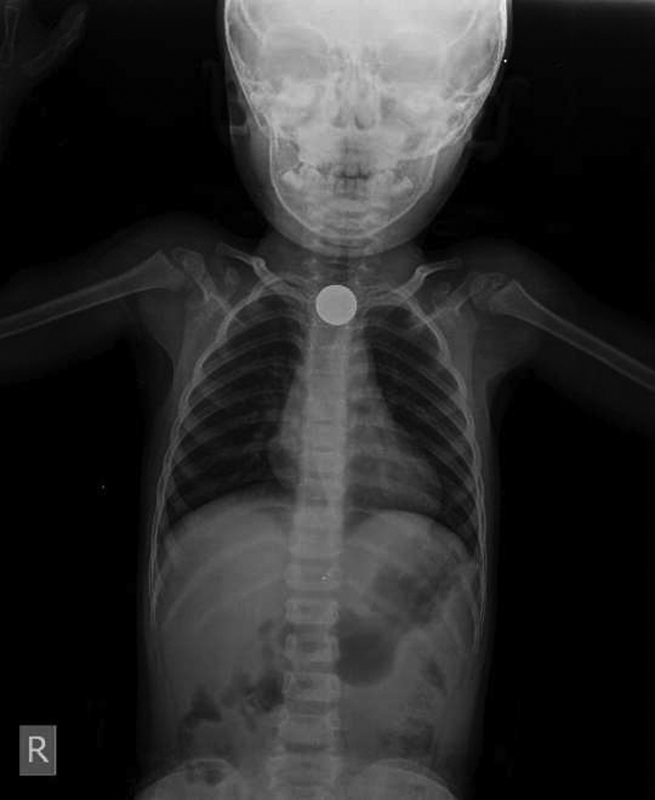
X-ray shows a coin in the first part of the esophagus.
